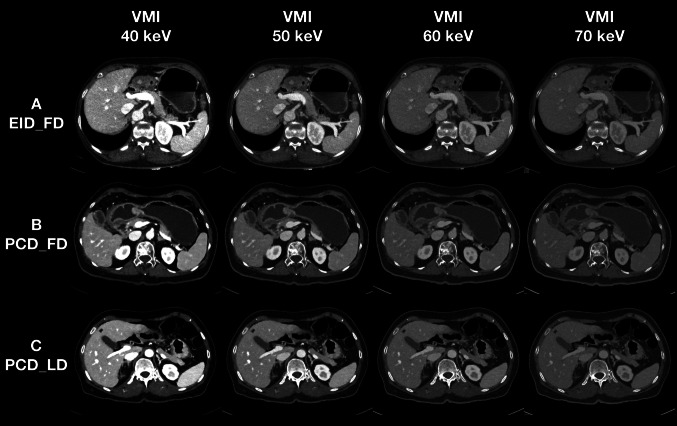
Representative cases for image quality assessment. Three matched cases of EID_FD, PCD_FD, PCD_LD scans of VMI at 40, 50, 60, and 70 keV for image quality assessment are presented with a window width of 800 HU and window level of 200 HU. (A) A 67-year-old female patient with a height of 1.59 m, a weight of 53.0 kg, and a body mass index of 20.94 kg/m^2^, who scheduled to undergo the contrast-enhanced abdominal CT scan for abdominal discomfort, on EID-CT with a full-dose protocol. (B) A 66-year-old female patient with a height of 1.56 m, a weight of 50.5 kg, and a body mass index of 20.74 kg/m^2^, who scheduled to undergo the contrast-enhanced abdominal CT scan before the endoscopy, on PCD-CT with a full-dose protocol. (C) A 66-year-old female patient with a height of 1.57 m, a weight of 50.0 kg, and a body mass index of 20.28 kg/m^2^, who scheduled to undergo the contrast-enhanced abdominal CT scan for suspicious gastric cancer, on PCD-CT with a low-dose protocol. The image noise increased with the decreasing of keV level of VMI, while the vessel sharpness became better with the decreasing of keV level of VMI. The image noise of EID_FD was higher than PCD_FD and PCD_LD, especially in VMI at 40 keV. The vessel sharpness was comparable between PCD_FD and PCD_LD. The PCD_FD and PCD_LD can provide better overall image quality than EID_FD. EID_FD = energyintegrating detector CT with full-dose protocol; PCD_FD = photon-counting detector CT with full-dose protocol; PCD_LD = photon-counting detector CT with low-dose protocol, VMI = virtual monoenergetic imaging, keV = kiloelectron volt
